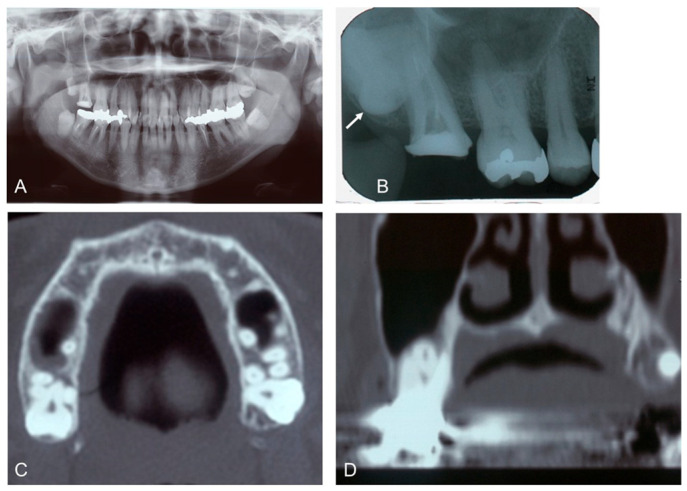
Initial X-ray images of the 46-year-old female patient. (A): Panoramic image. Note: impacted wisdom teeth (#1, 16, 17, 32). (B): Dental image (#1, 2, 3, 4). Arrow indicates horizontally impacted tooth (#1). (C): CT (axial image). (D): CT (coronal image) showing root apex of upper right third molar (#1) placed between buccal and palatal roots of upper right second molar (#2).
